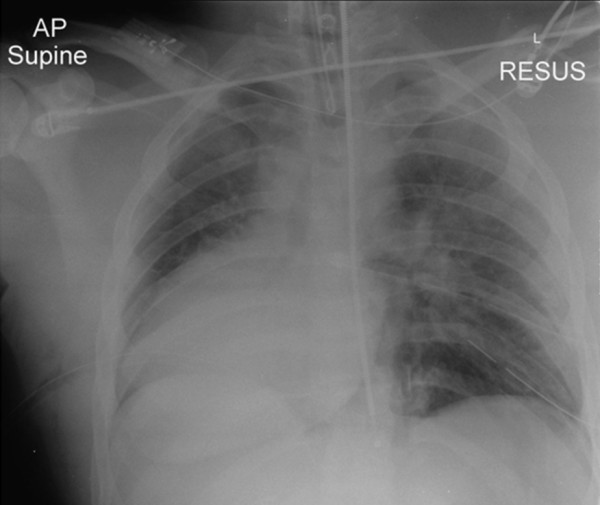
Plain supine AP chest radiograph showing a prominent, right-sided cardiac silhouette ('boot shaped'); bilateral pulmonary contusions; rib fractures; endotracheal and tube thoracostomies. With the benefit of hindsight there is the suggestion of a left-sided pneumopericardium surrounded by a faint pericardial contour.
